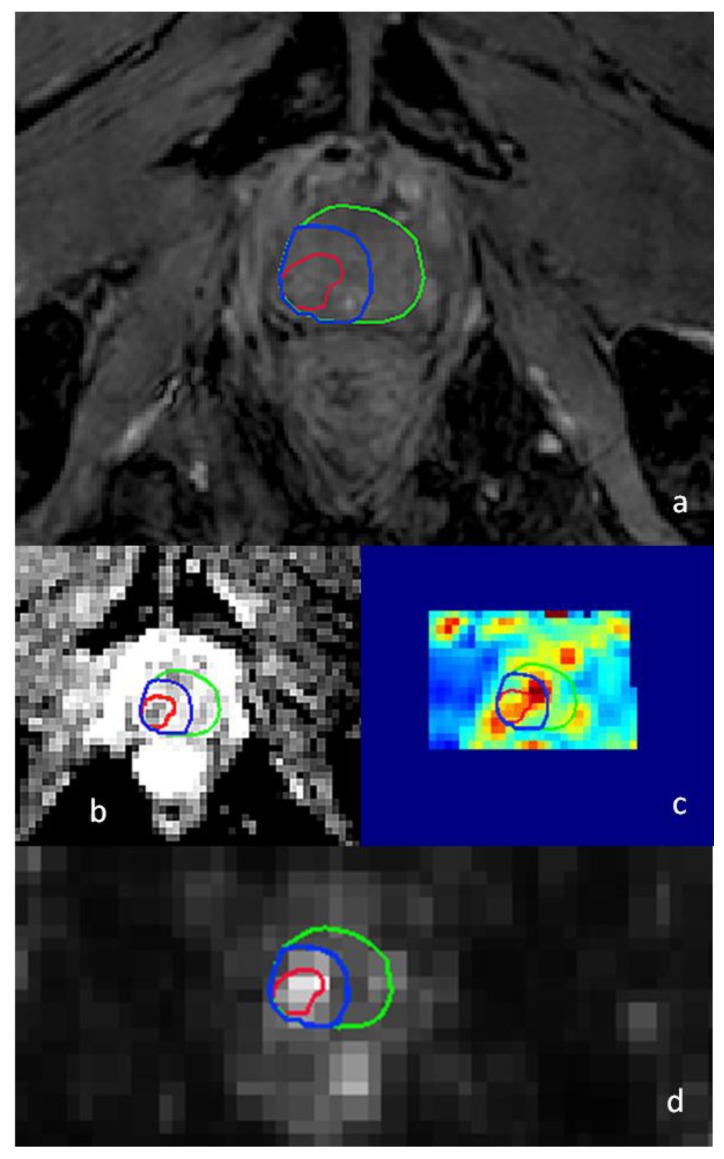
Recurrent prostate cancer lesion on diagnostic 3-T multiparametric magnetic resonance imaging (mp-MRI) (a–c) and prostate-specific membrane antigen positron-emission computed tomography (PSMA-PET/CT) (d). The suspect lesion is visible in the right peripheral zone of the apex. Delineations of the prostate (green), gross tumor volume (GTV, red), and clinical target volume (CTV, blue) are displayed. (a) T2-weighted MRI; (b) apparent diffusion coefficient (ADC) map of diffusion-weighted imaging (DWI)-MRI; (c) K-trans map of dynamic contrast-enhanced (DCE)-MRI; (d) ^68^Ga-PSMA-PET/CT.
